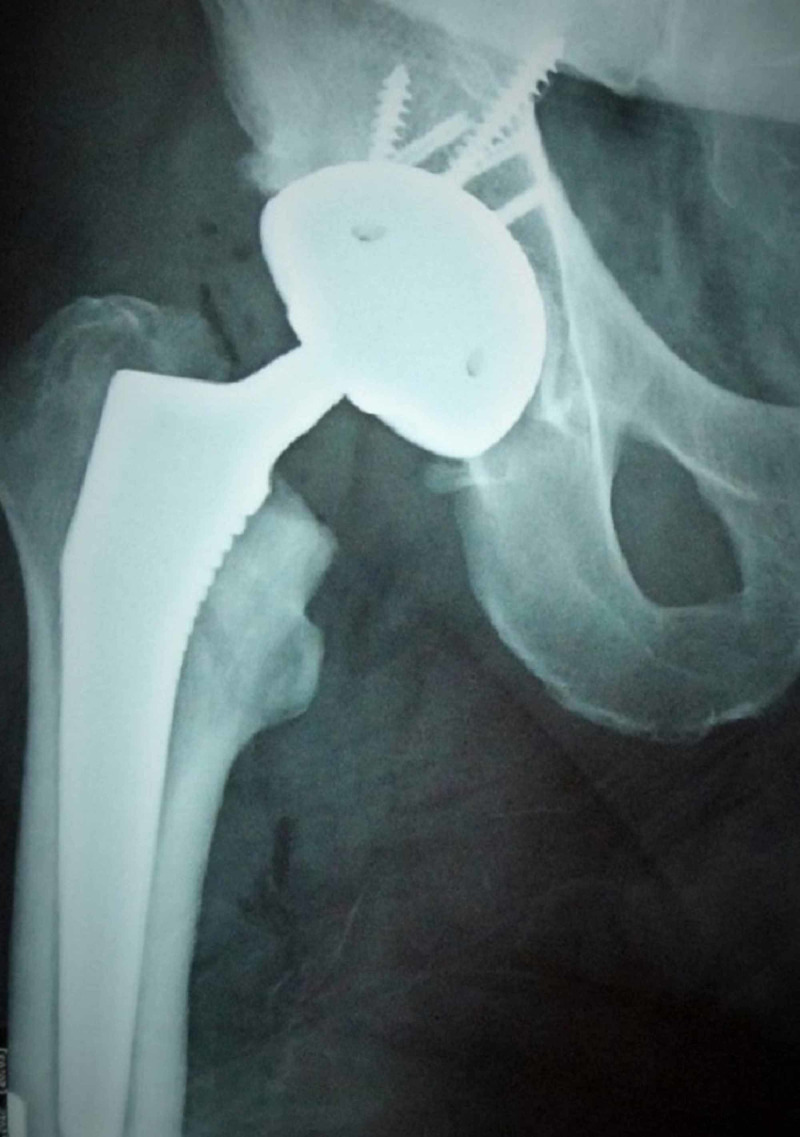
Radiograph of the same patient three months postoperatively
